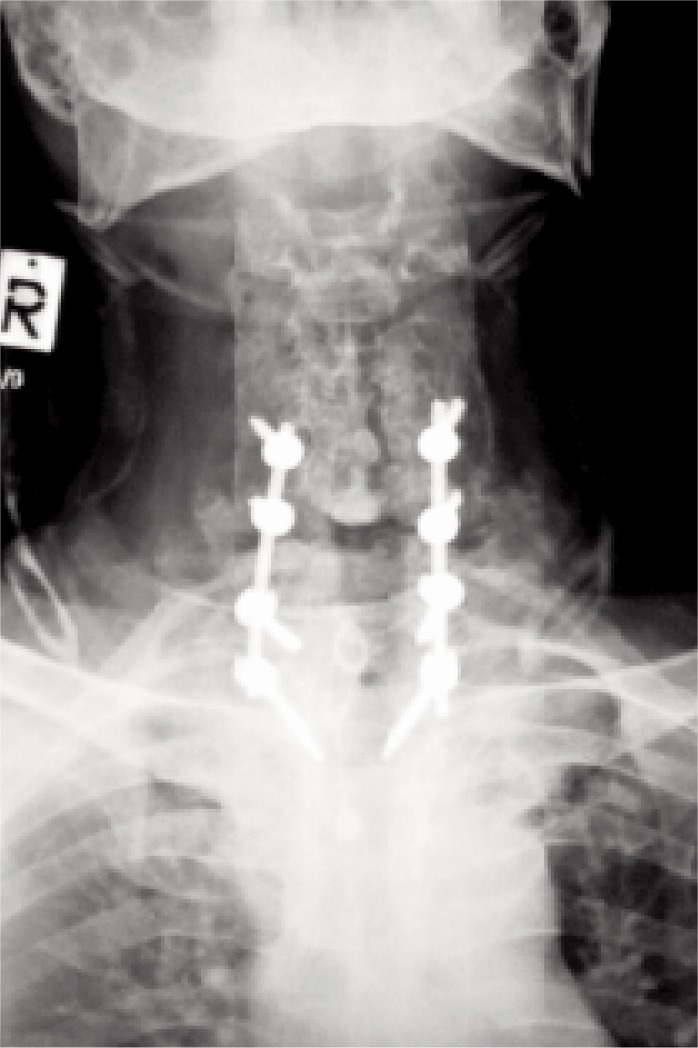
Radiograph following posterior spinal instrumentation and fusion of C4/C5/C7/T1.
